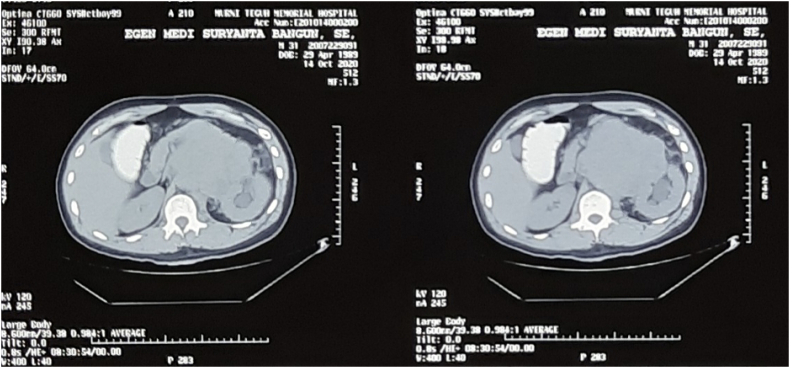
CT scan with contrast prior to patient first orchiectomy (right testis) and before BEP administration.
